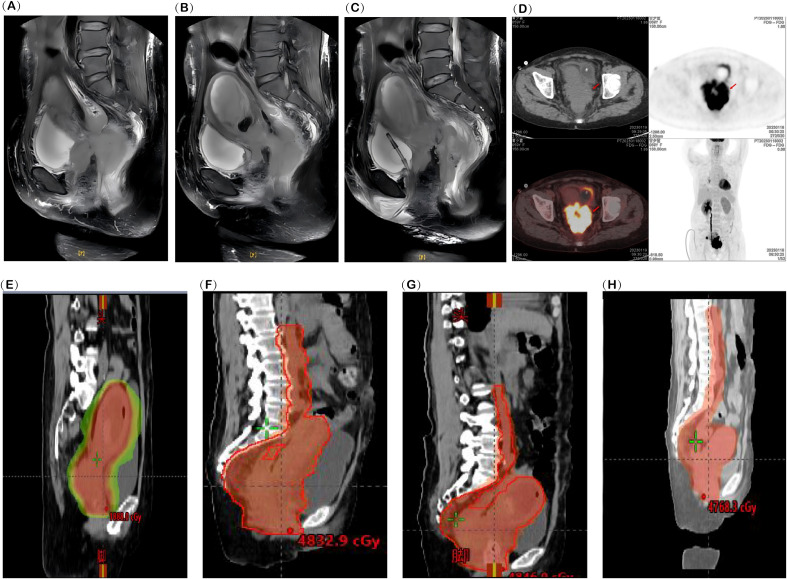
Baseline clinical presentation, multimodal imaging findings, emergency hemostatic radiotherapy, and adaptive radiotherapy planning during treatment. (A) Gynecological examination demonstrating a large exophytic cervical mass with active vaginal bleeding. (B, C) Baseline pelvic magnetic resonance imaging (MRI) demonstrating a bulky cervical tumor involving the uterine body and lower third of the vagina, with invasion of the rectum and posterior bladder wall. (D) Baseline positron emission tomography–computed tomography (PET–CT) showing intense metabolic activity in the primary cervical lesion with pelvic and para-aortic lymph node metastases, without evidence of distant organ metastasis. (E) Emergency hemostatic intensity-modulated radiotherapy (IMRT) plan delivered for rapid bleeding control. (F) Definitive pelvic external radiotherapy target delineation and dose distribution. (G, H) Adaptive replanning during treatment based on tumor regression and anatomical changes, demonstrating progressive reduction of irradiated target volume during serial treatment adaptation.
